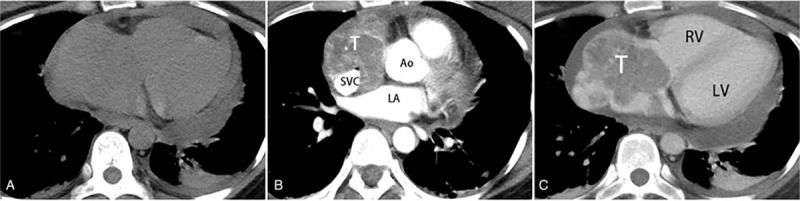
CT features of a malignant peripheral nerve sheath tumor in right atrium. (A) A lobulate mass (average CT value: 44 HU) locates in the RV with bilateral mild plural effusion and moderate pericardial effusion on CT plain scan. (B) At arterial phase, the mass is heterogenous and markedly enhanced (average CT value: 104 HU) with SVC being involved. (C) At the venous phase, the lesion tends to wash-out (average CT value: 78 HU). Note: T = tumor, SVC = superior vena cava, Ao = aorta, LA = left atrium, LV = left ventricle, RV = right ventricle, CT = computed tomography.
